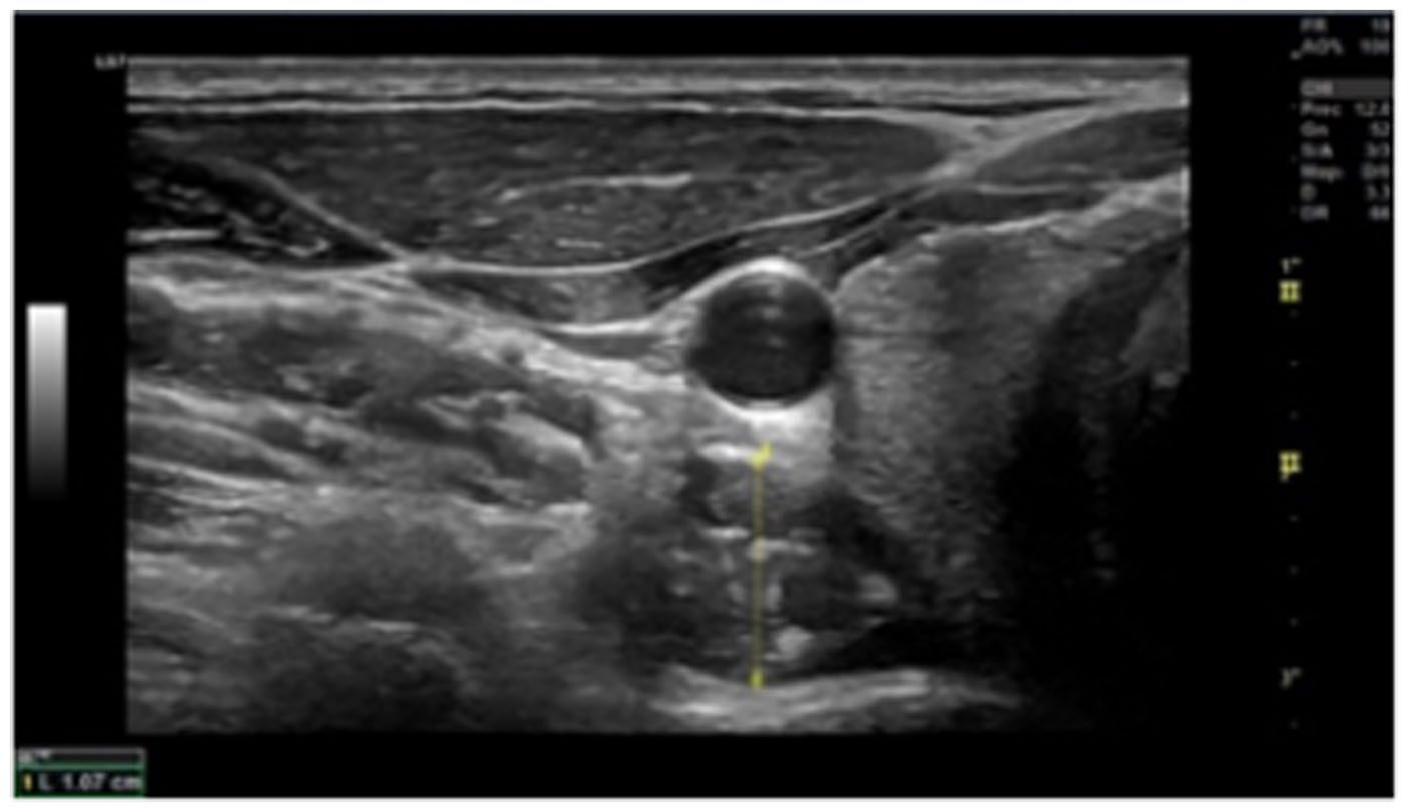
Ultrasound image of the longus colli at C6 (transverse view). The probe is positioned on the interface between the Lcol and the pre-fascial tissue encircling the carotid artery, as well as the midpoint of the ventral surface of the C6 vertebral body.
